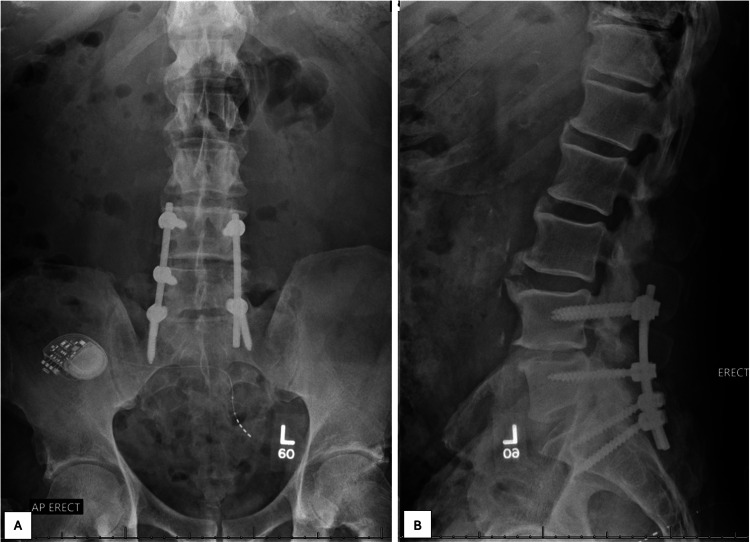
Plain film standing radiographs of the lumbar spine obtained two months postoperatively, which demonstrated no concerning osseous findings, good alignment, and stable appearance of posterior instrumentation. Figure 8A demonstrates an anterior-to-posterior radiograph of the lumbar spine, while Figure 8B demonstrates a lateral radiograph of the lumbar spine. The patient also reported resolution of his back and leg pain at this point.
